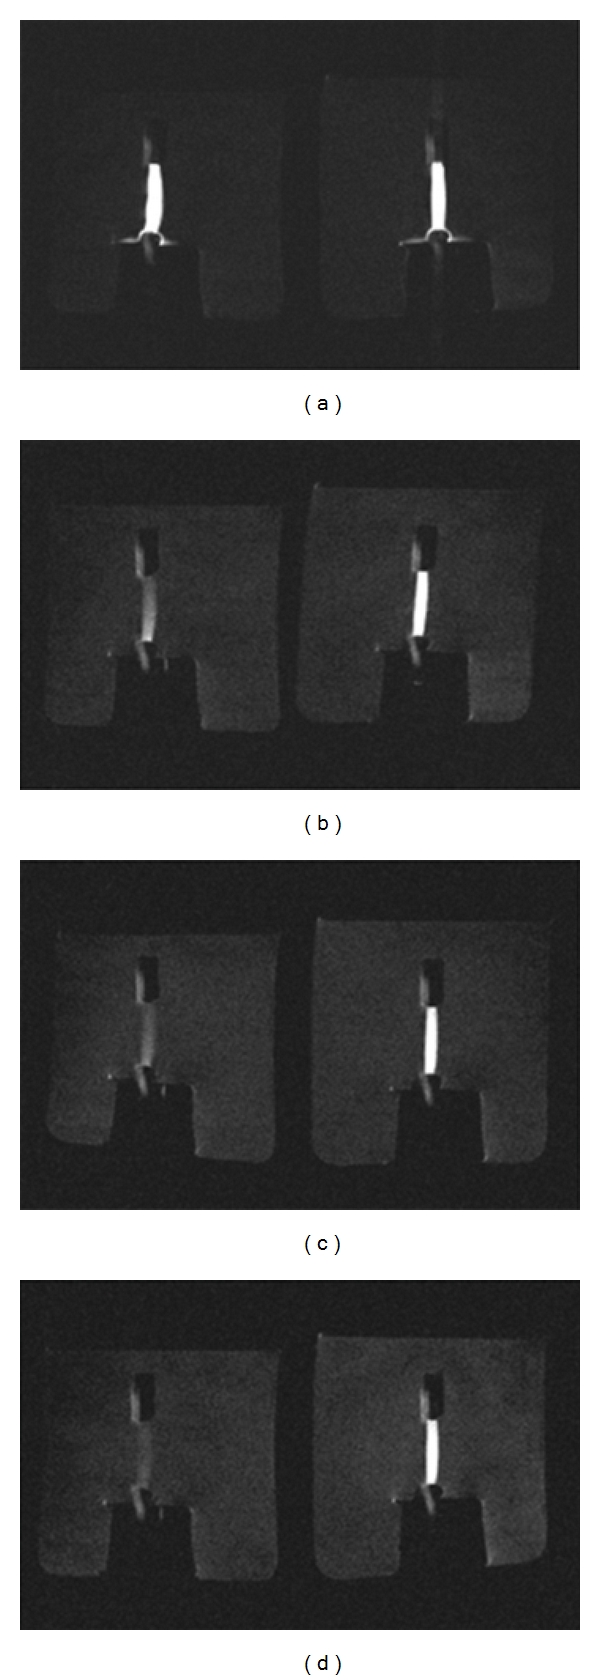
Magnetic resonance image montage of the progression of contrast media diffusion through a dialysis cassette at *t* = 0, 329, 407, and 1489 minutes after injection, starting from top-left row, ending at bottom right. At 24 hours, the Gd DTPA-BMA (left fixture) has leaked out, while the HWO1-147 (right fixture) is relatively well-retained inside its cassette.
